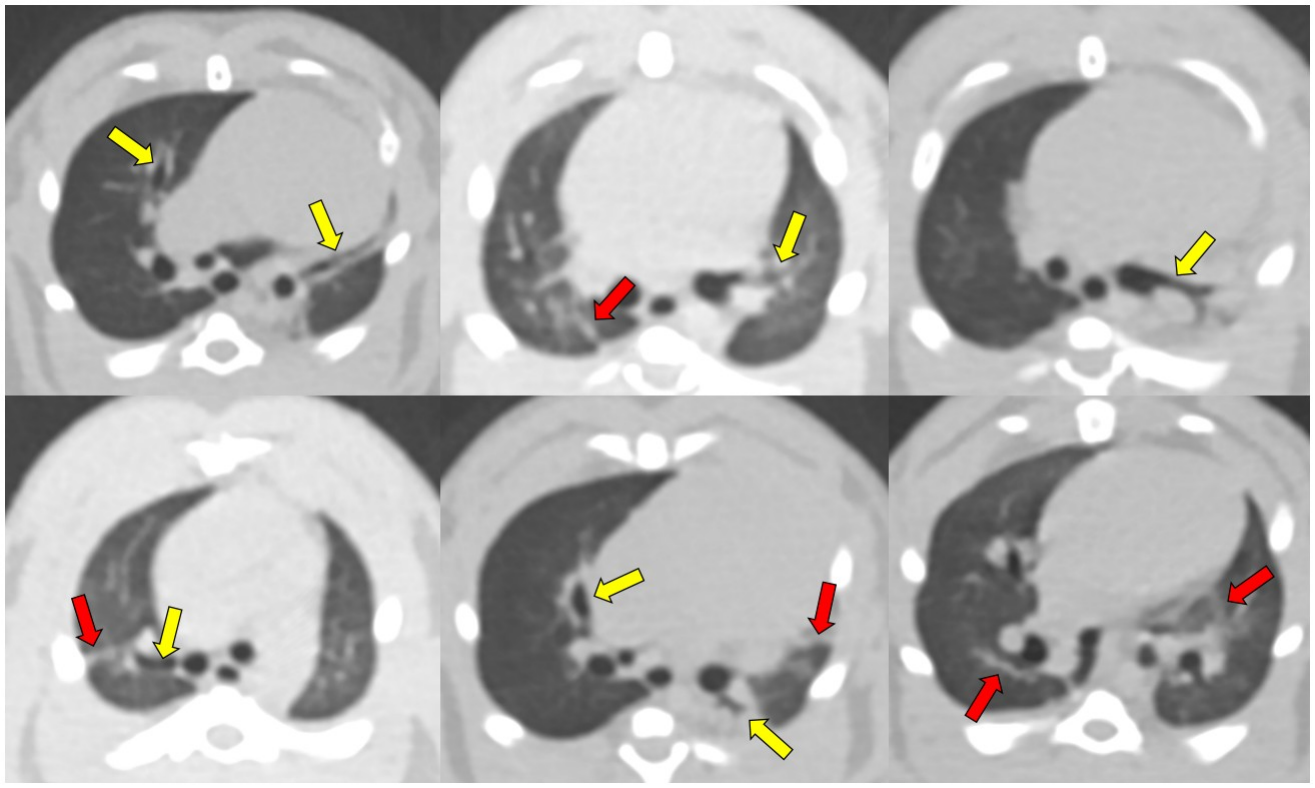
Representative CT images of bronchiectasis (yellow arrows) and linear densities (red arrows) correlated with lung fibrosis at 8, 26, and 52 weeks after PHMG exposure.
